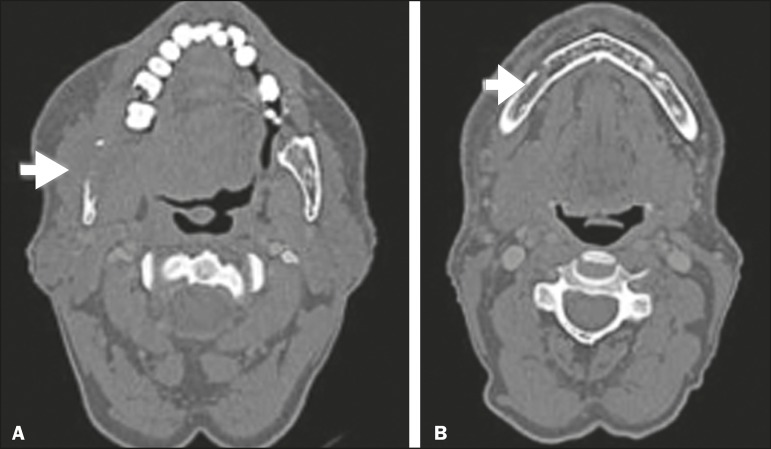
Contrast-enhanced CT scan of a 63-year-old male with squamous cell
